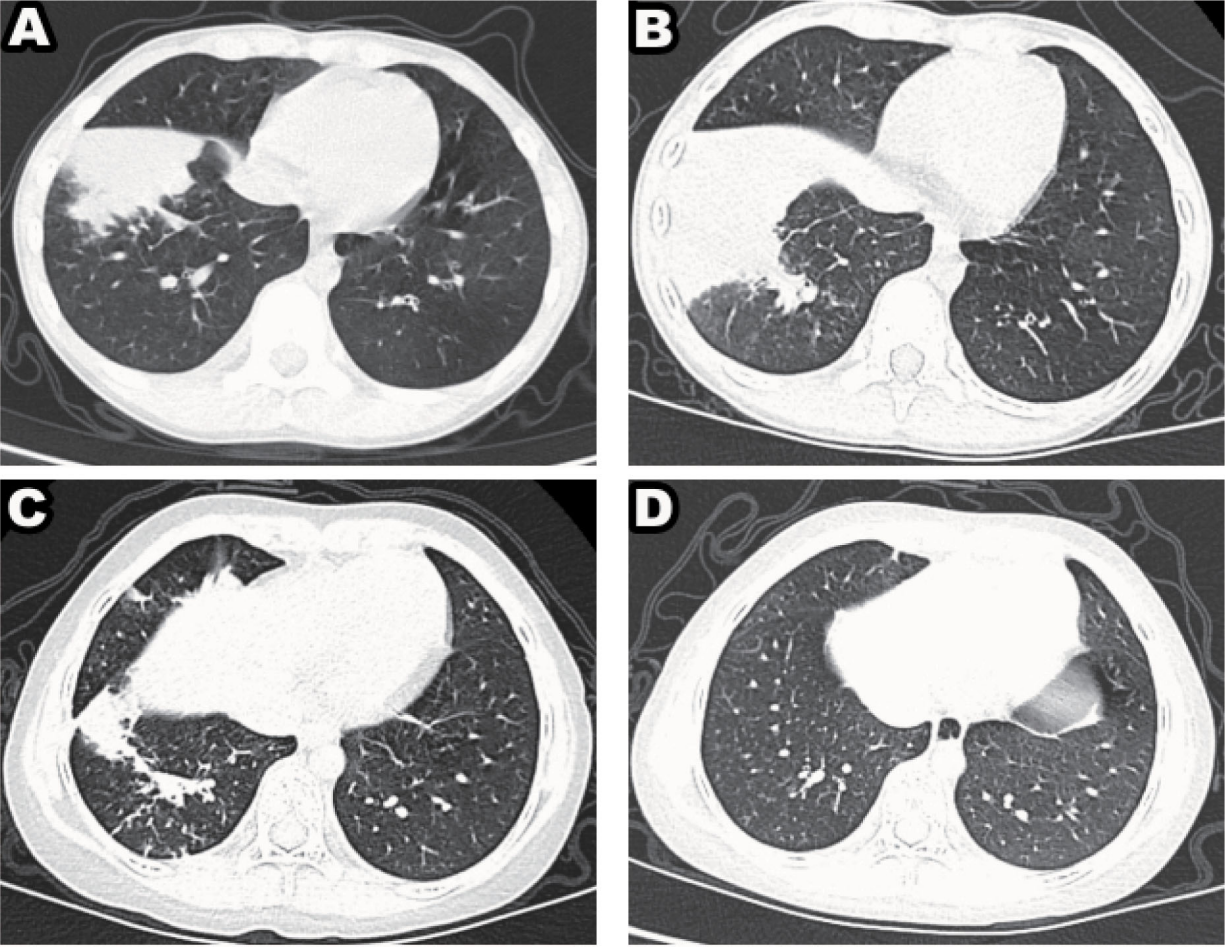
Mycoplasmal pneumonia in a child (male, 10 years old), mainly manifested as large patchy consolidation with poor prognosis, seven days later, CT image shows the extent of distribution increased (A, B). Mycoplasmal pneumonia in a child (male, 12 years old) with favorable prognosis, seven days later, CT image shows the lesions to be absorbed (C, D).
